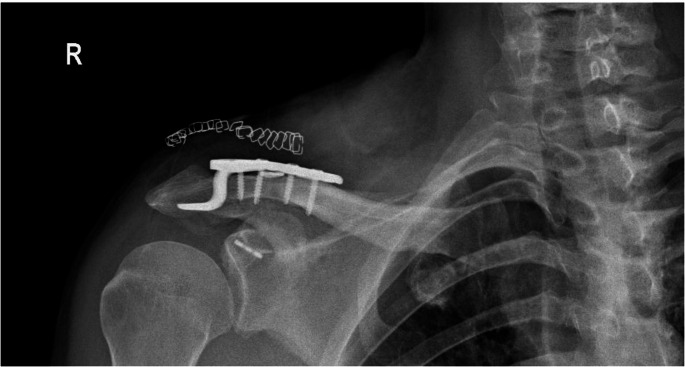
Postoperative radiograph of the same patient demonstrating fixation using a TightRope device in combination with a hook plate. Plate removal was typically scheduled approximately six months postoperatively but was performed earlier in cases where patients reported significant plate-related discomfort or irritation
